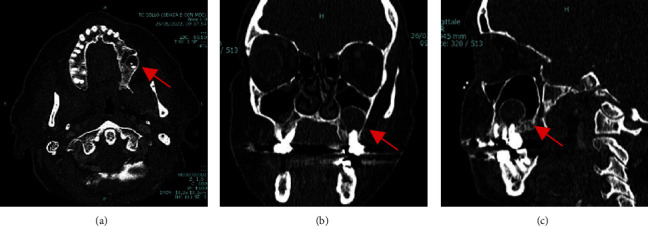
Case 3: preoperative CT scan showing round, hypodense lesion associated to the roots of a molar teeth, protruding in the left maxillary sinus (red arrows). (a) Axial view, (b) coronal view, and (c) sagittal view.
